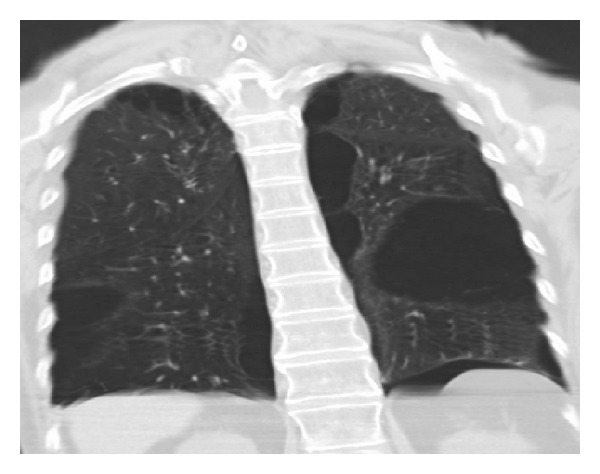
Chest CT coronal view showing bilateral lung bullae more than one-third of the left hemithorax.
